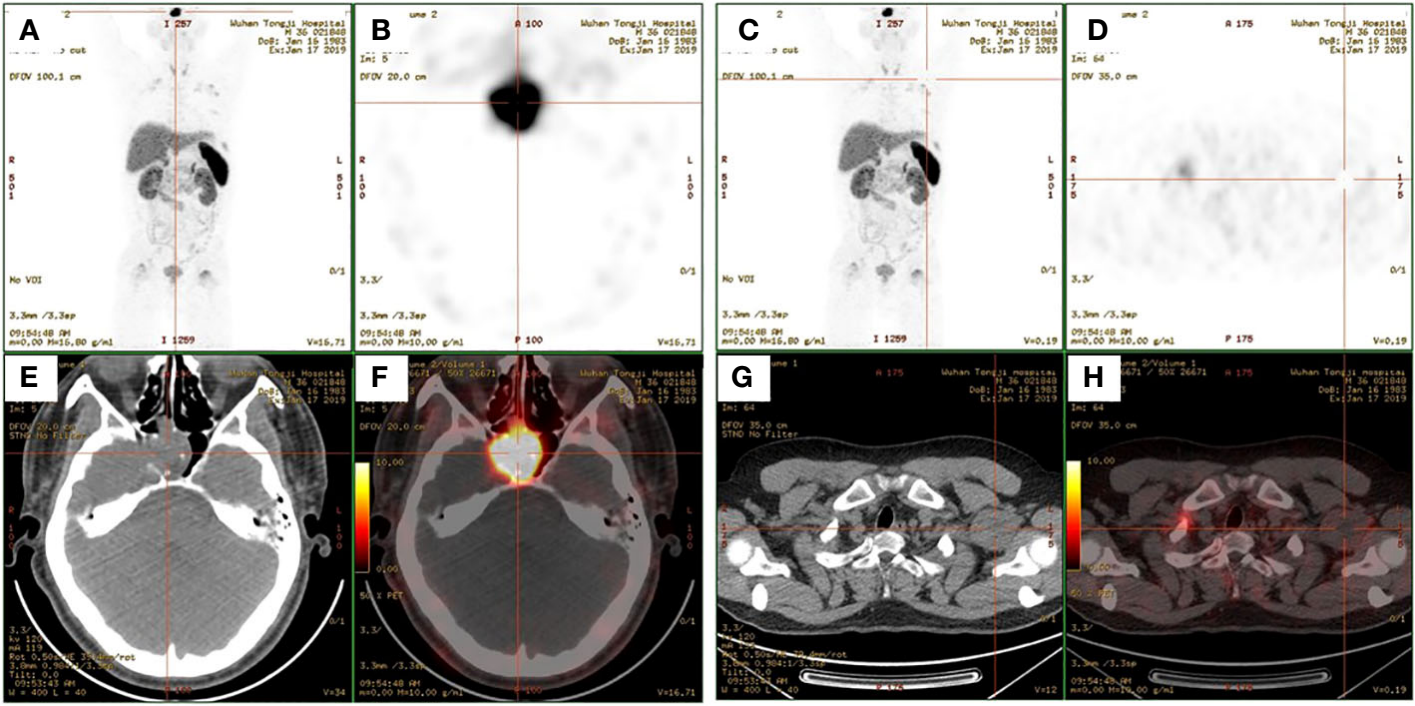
(A, C) Maximum-intensity projection images of the patient. (B, D) Axial views of the sphenoid sinus tumor (B) and the axillary tumor (D) in 68-gallium 1,4,7,10-tetraazacyclododecane-1,4,7,10-tetraacetic acid octreotate (Ga-68-DOTATATE) positron emission tomography (PET). (E, G) Axial views of the sphenoid sinus tumor (E) and the axillary tumor (G) in CT. (F, H) Fusion images.
